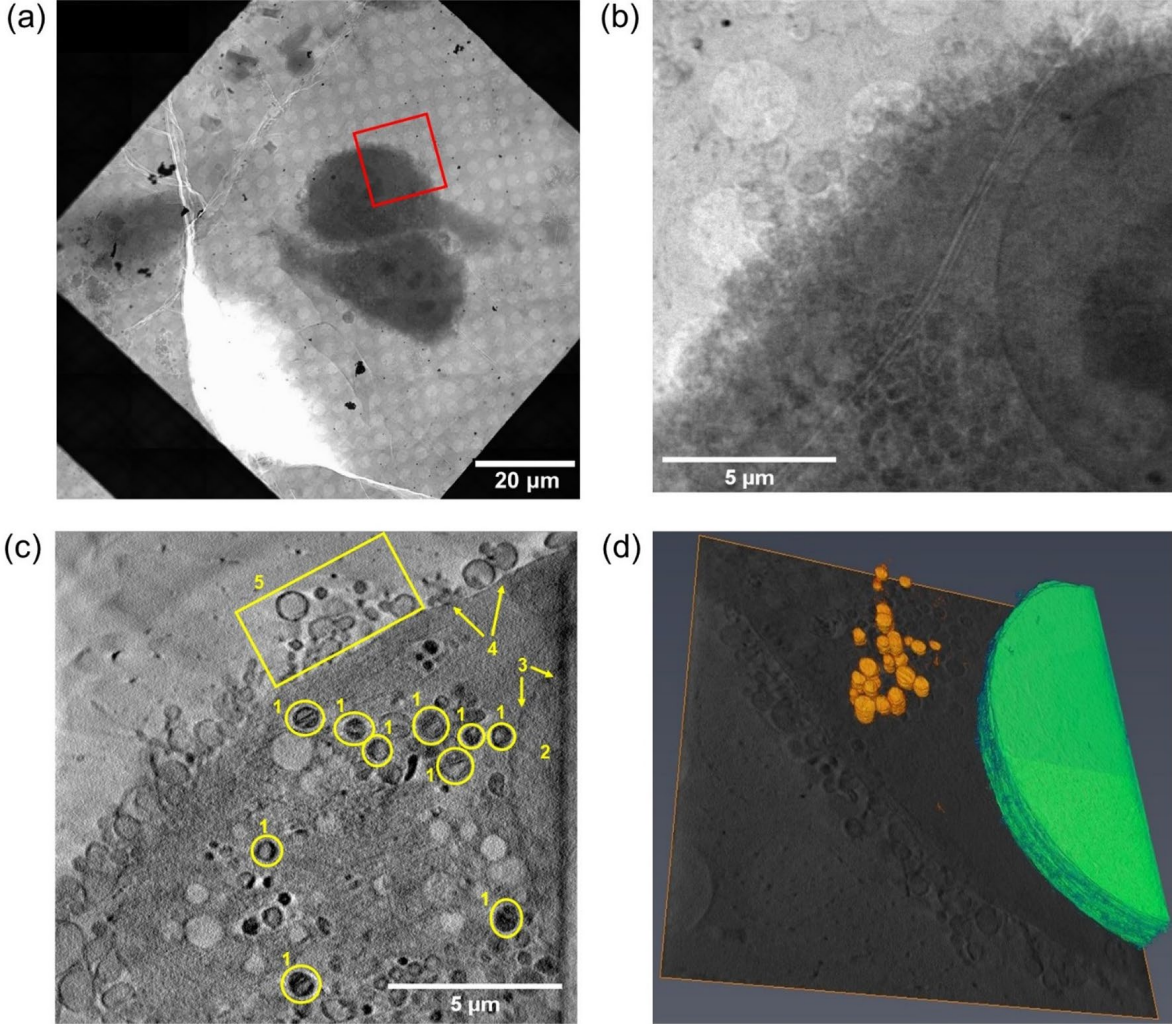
Cryo-SXT images of cryogenically-fixed PC3 prostate cancer cells grown on carbon–gold TEM grids (24 h) and treated with 1 µM of 1 for 2 h, followed by 10 min irradiation with blue light (*λ* = 465 nm) and 24 h recovery in complex-free medium: a X-ray mosaic image of a 100 × 100 μm^2^ area of the grid; b X-ray projection image collected from a 16 × 16 μm^2^ area of interest (marked as a red square) in a after exposure to soft x-rays (8 s, 500 eV); c 2D projection of the reconstructed tomogram obtained from the same area (in b); showing 1 = mitochondria, 2 = nucleus, 3 = nuclear membrane, 4 = plasma membrane, 5 = vesicles; d 3D segmented tomogram analysed in SuRVoS and visualised in Amira; showing mitochondria (orange) and nucleus (green). Images were generated using ImageJ (a) [40], IMOD (b, c) [33, 34] and SuRVoS and Amira imaging softwares (d) [34, 36]
